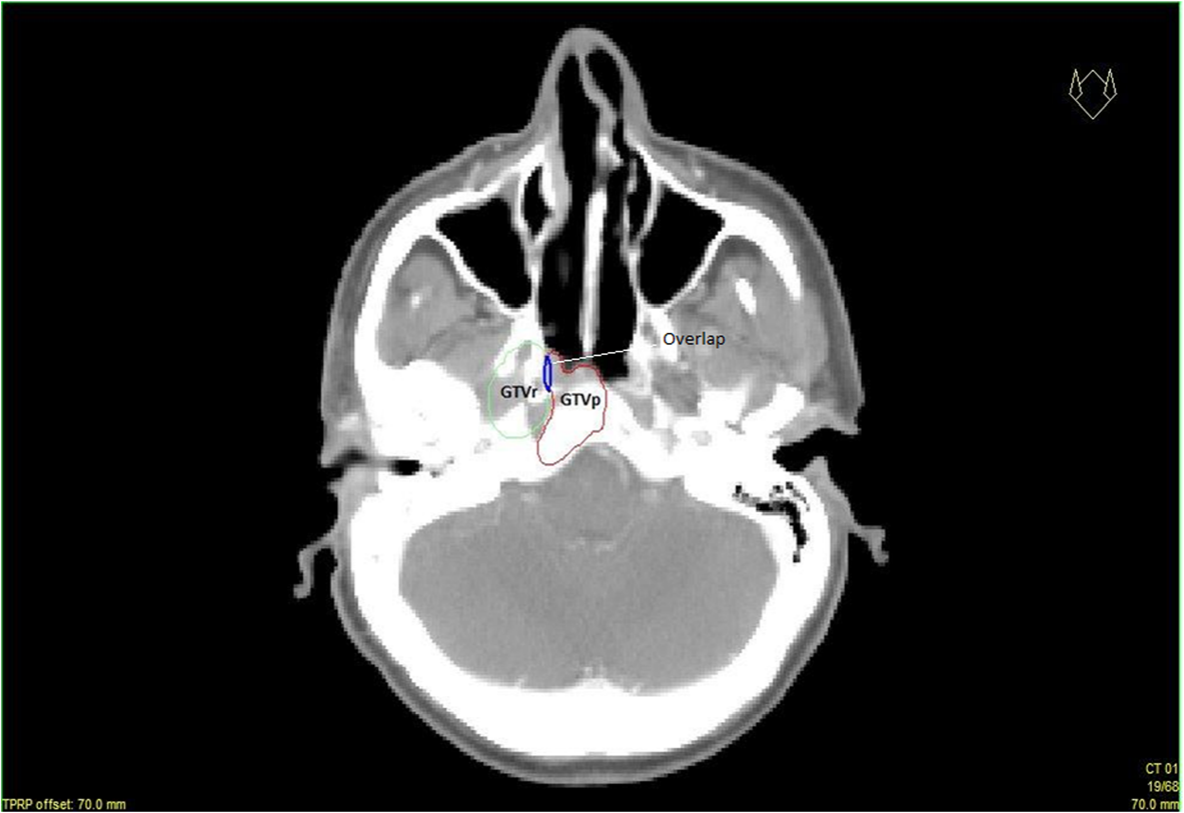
Fusion display of primary (gray) and recurrence (pink) CT images
